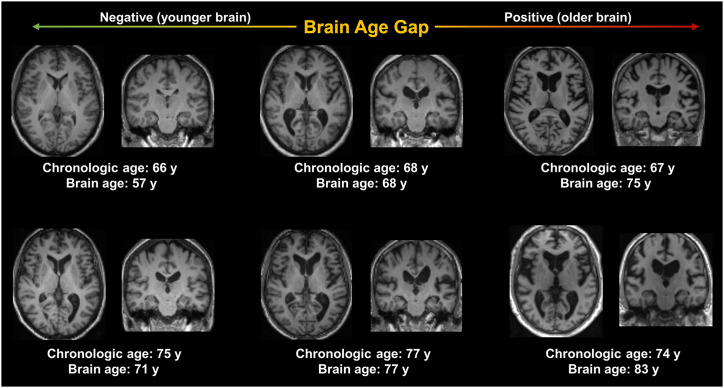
Chronologic and structural MRI brain ages of 6 patients with MCI. Age effects are distinctly apparent on structural MRI, for example, in enlargement of ventricles, as well as general cortical and subcortical atrophy. BAG captures acceleration of brain aging, for example, due to neurodegenerative disease, and can provide useful information for disease and therapy monitoring. Brain age was estimated using our models previously implemented in Doering et al. (19) for our previous publication. Same slice is depicted for all scans.
